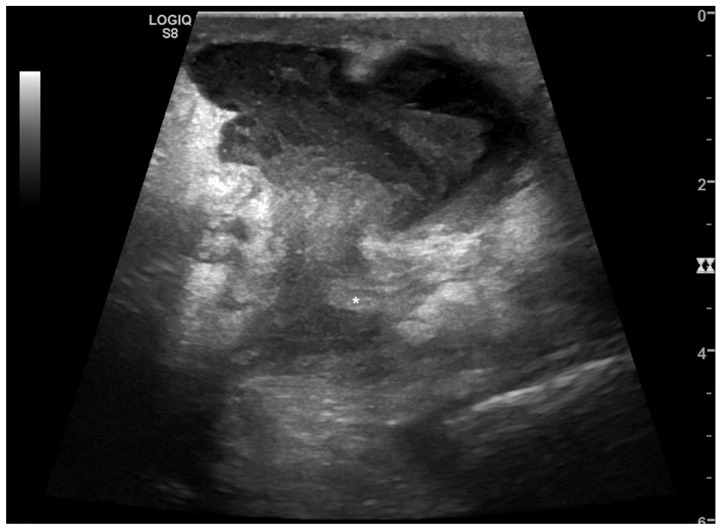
Black where it should not be. A 60-year-old male patient who underwent laparotomy gastrectomy with fever and abdominal pain. At ultrasound, a pluriloculated fluid collection was detected in the abdominal wall with a fistulous connection in the peritoneal cavity. No free fluid in the abdomen was detected. A suspected diagnosis of infected collection along the laparotomy suture was formulated. Final diagnosis: abscess along the laparotomy suture with peritoneal fistulous connection.
